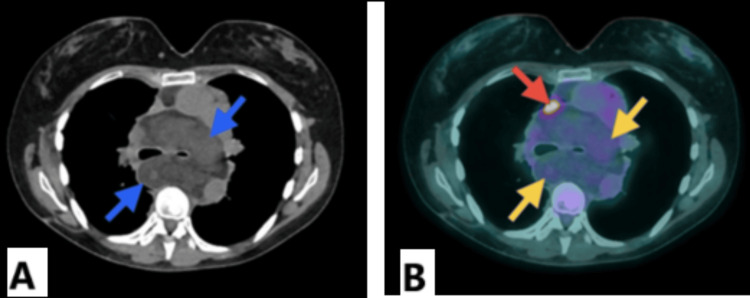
(A) Axial CT (soft-tissue window) showing a well-defined, multilobulated mediastinal mass with predominantly fat density (blue arrows). (B) Axial PET/CT fusion showing alternating normometabolic and mildly hypermetabolic areas (yellow arrows) (SUVmax 2.54) and marked hypermetabolism (SUVmax 7.09) along the SVC (red arrow), associated with an endoprosthesis CT: computed tomography; PET: positron-emission tomography; SVC: superior vena cava
